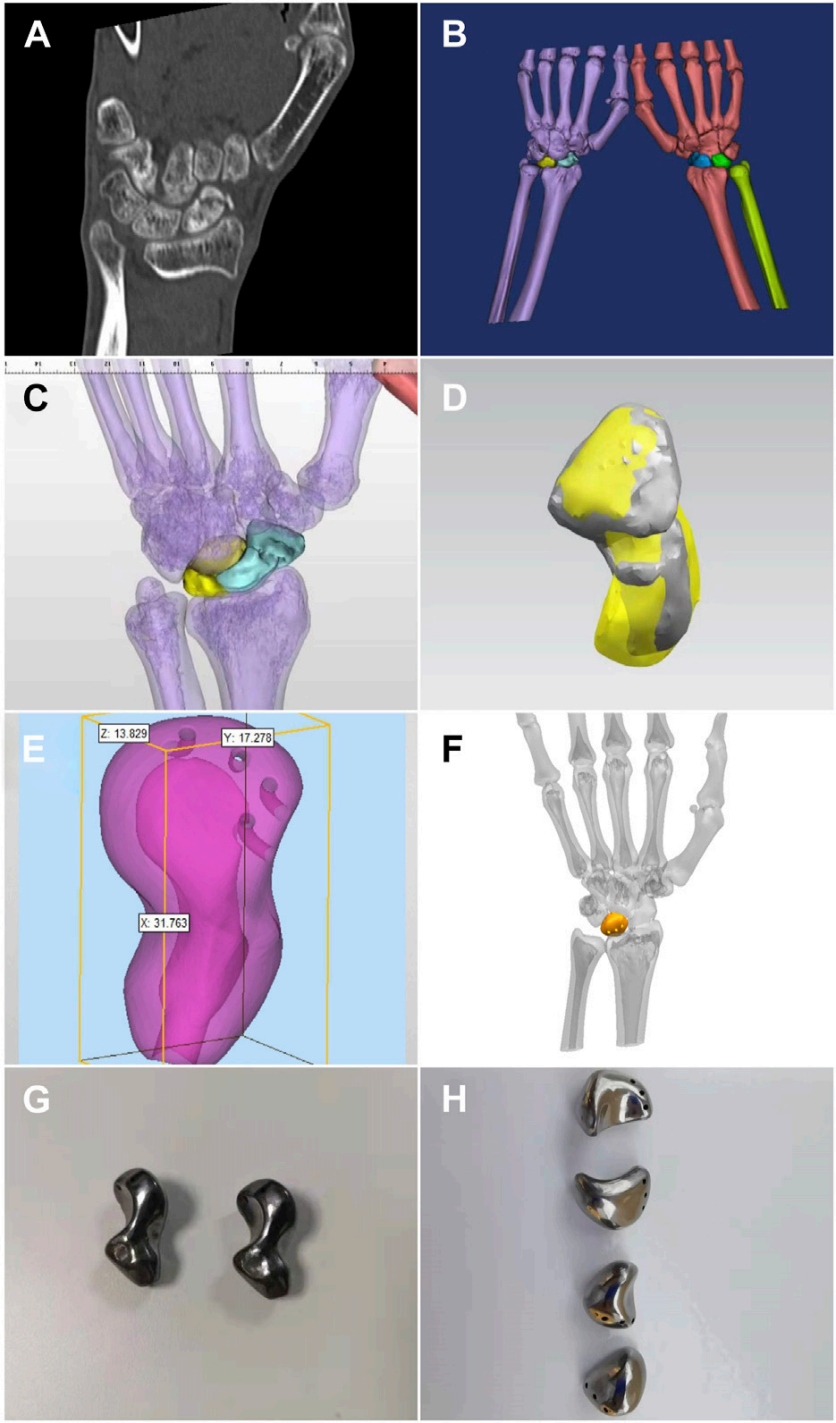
Carpal prosthesis design process. (A) Preoperative CT thin-layer scan; (B) Bilateral carpal 3D model reconstruction; (C) Mirror image of the healthy scaphoid coincides with the affected side after flipping; (D) Frontal view of the 3D model of the scaphoid prosthesis design; (E) Size measurement of the scaphoid prosthesis; (F) Frontal view of the 3D model of the lunate prosthesis design; (G) 3D printed tantalum scaphoid prosthesis; (H) 3D printed tantalum lunate prosthesis.
